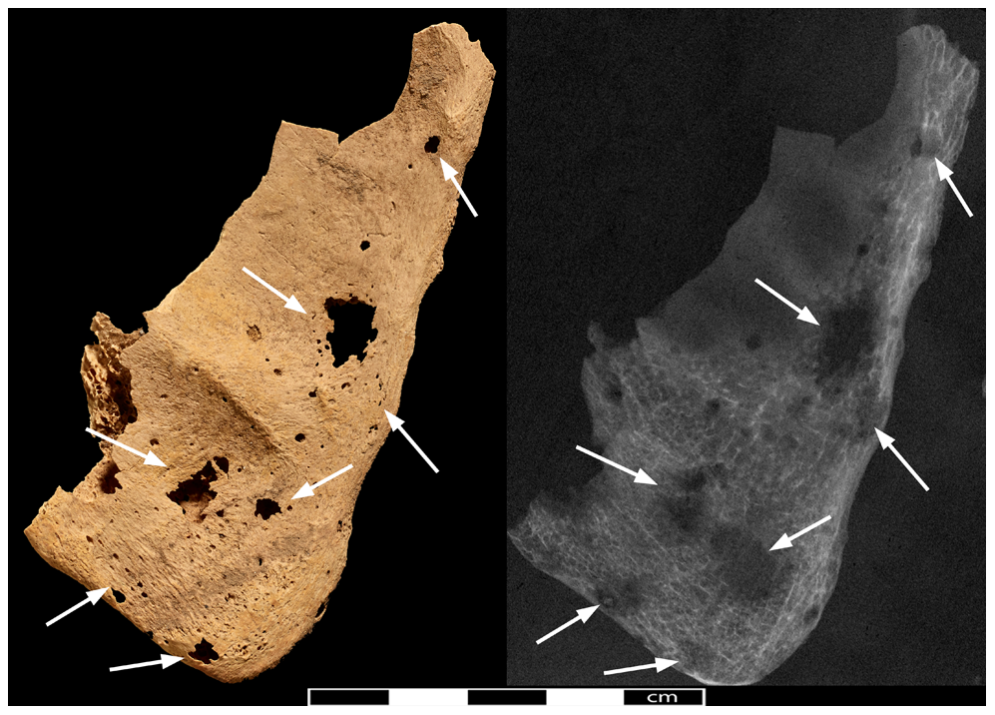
Pathological lesions in the right scapula. Photo- and radiograph of the right scapular blade (view from anterior, arrows indicate location of lytic lesions).
